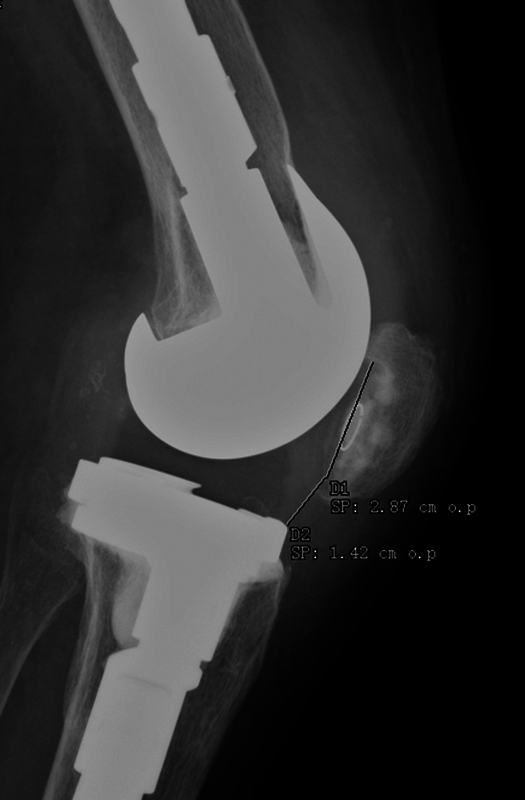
Lateral radiograph of the knee showing the preoperative Caton-Deschamps index 0.49.
